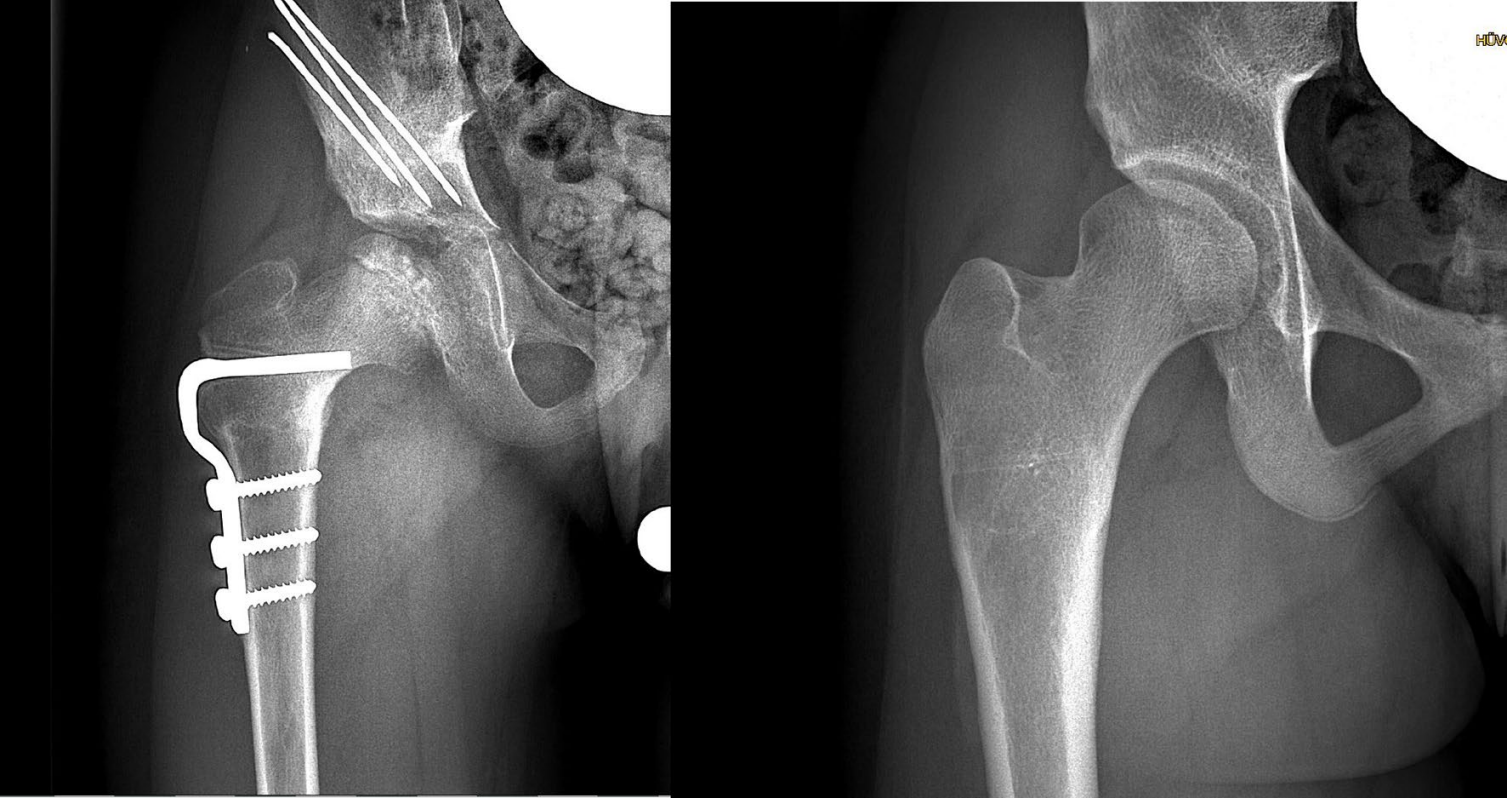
Anterior–posterior X-rays of an 8-year-old girl with LCPD with deterioration of pelvic coverage 7 years postoperatively
